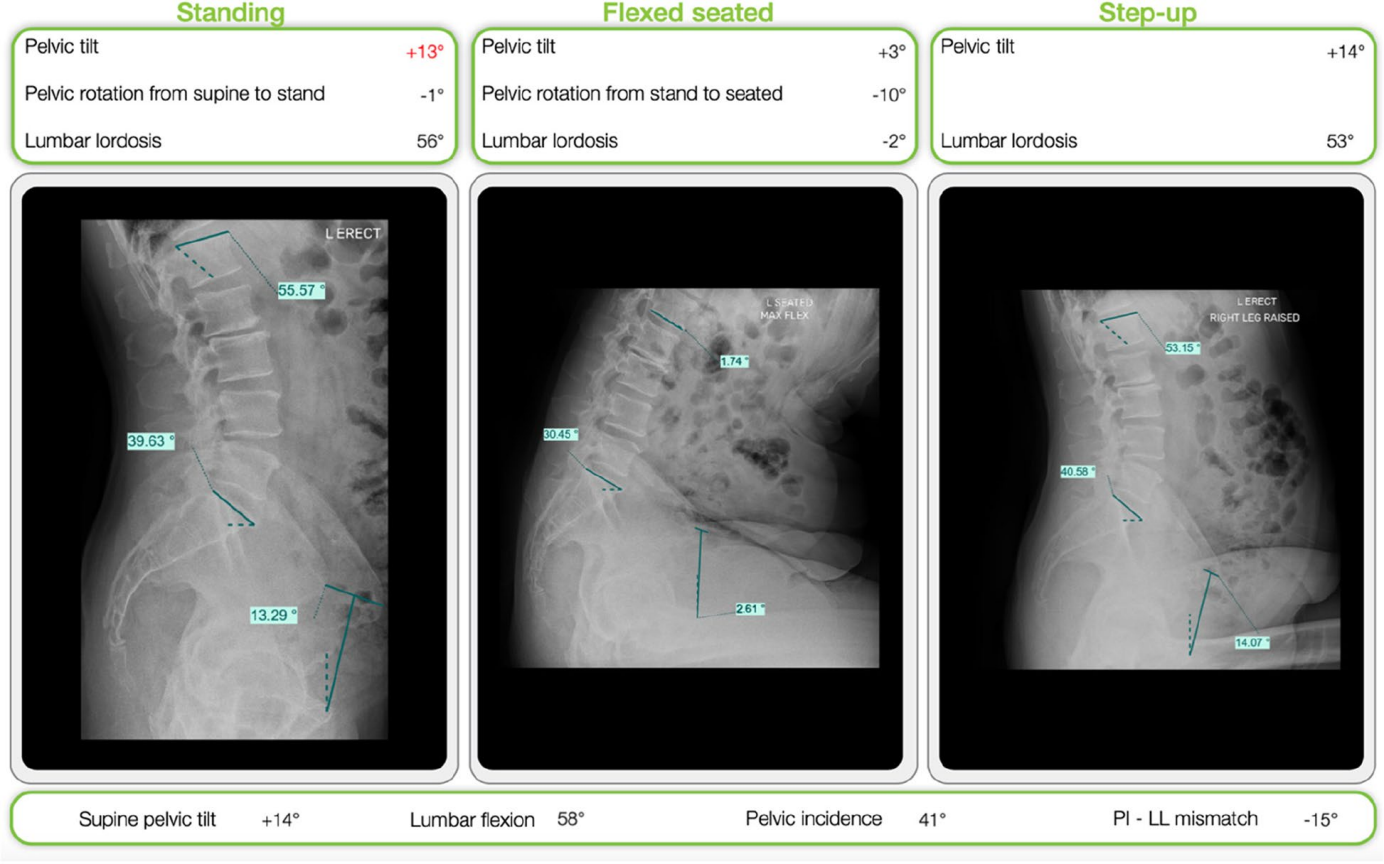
Lateral radiographs evaluated on OPSinsight with pelvic parameters shown
